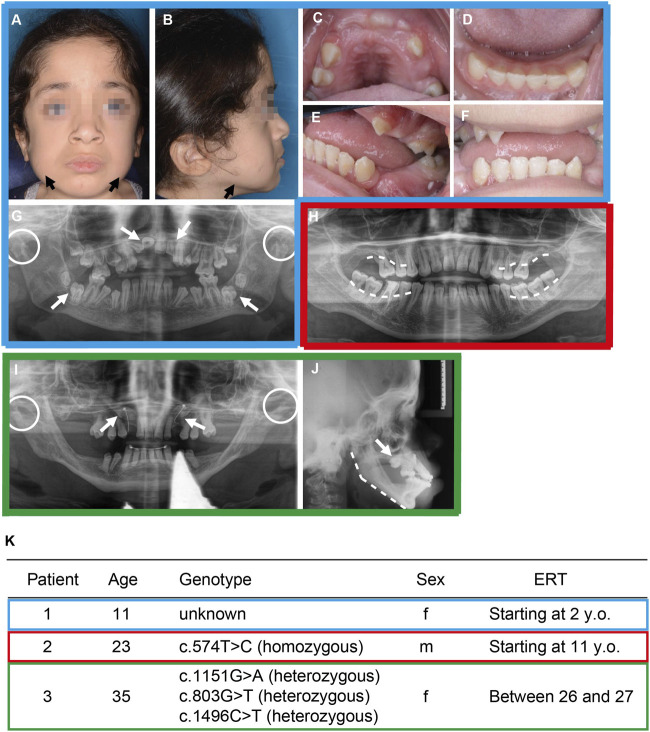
Craniofacial and dental pathologies of patients with Mucopolysaccharidosis Type VI. (A–G) Patient #1 at 11 years treated with ERT (Naglazyme, BioMarin Pharmaceuticals) starting from the age of 2. (H) Patient #2 at 23 years treated with ERT starting at the age of 11 years. (I–J) Patient # 3 at 35 years treated with ERT between the age of 26 and 27. (A–B) Extraoral photographs display the MPS VI–typical facial coarsening with mandibular bone exostoses at the jawline (black arrows). (C–F) Intraoral photographs show gingival hyperplasia and delayed anterior teeth eruption, which normally erupts in the age between 6 and 9 years. (G) Panoramic radiograph image showing displaced and retained incisors and dentigerous cysts of the first mandibular molars (white arrows). Bleeding on probing was observed in periodontal screenings. Furthermore, condylar hypoplasia is marked with white dotted circles. (H) Panoramic radiograph image reveals progressive maxillar and mandibular horizontal bone loss (white dashed lines). In periodontal screenings, probing depths over 6 mm were measured. Teeth 37 and 47 were extracted afterward due to increased mobility. Mandibular condyles are not shown in this image. (I) Panoramic radiograph image showing retained cuspids and orthodontic traction chains (white arrows). Condylar hypoplasia is marked with white dotted circles. (J) Lateral cephalometric radiograph displaying severe vertical facial growth including flat mandibular angle (white dashed line). Retention of the cuspids is shown from a lateral view (white arrow). (K) General information of the three patients including genomic modification within the ARSB gene. Here, (C)574T>C, corresponding to p. C192R, and (C)1151G>A (p.S384N) are pathological (Lin et al., 2008; Tomanin et al., 2018). The mutation (C)1496C>T (p.L499P) is probably also pathological, since an L498P substitution impairs the a-helix structure of ARSB and thus probably its function. (Litjens et al., 1996). However, we additionally identified a previously unknown mutation c803G>T (p.G268V), which was not previously described in the literature (Panel 1K).
